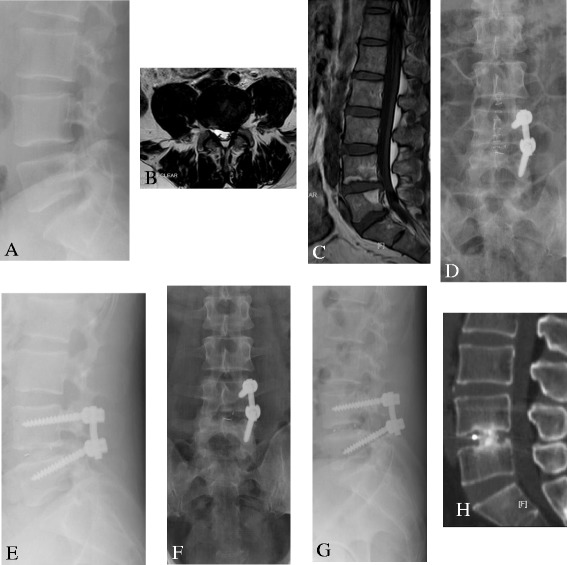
a, b A 46-year-old man suffered from lower back pain with radiation to the left leg. MR showed that the intervertebral disc for L4/L5 was herniated, and the left nerve root was compressed. d, e. The patient was treated with unilateral PLIF using a single cage supplemented with unilateral pedicle screws via the right side. f–h The radiograph and CT scan at 1-year follow-up showed bony trabeculae bridging the fusion level
